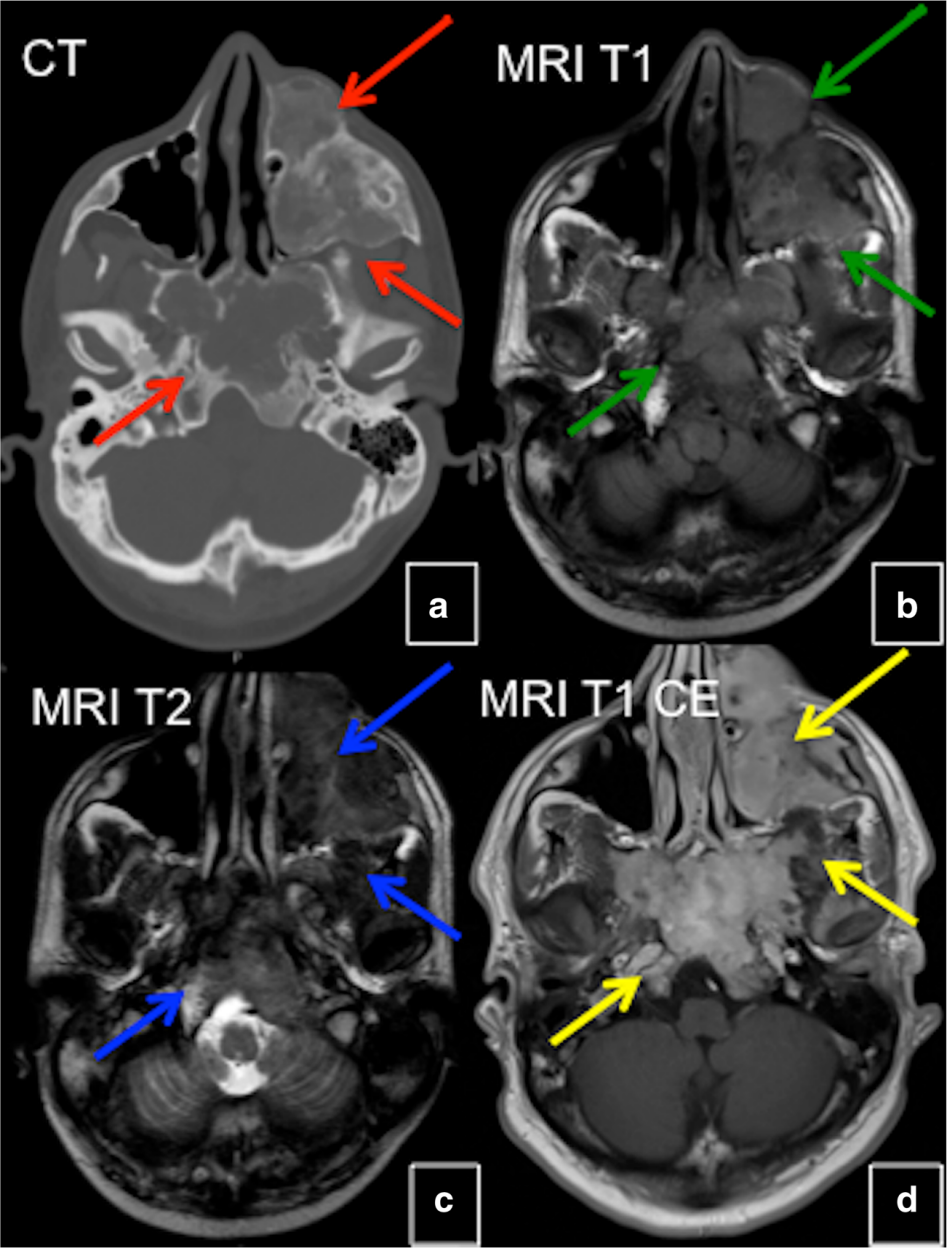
CT and MRI in craniofacial fibrous dysplasia (FD). a CT demonstrates mixed sclerotic FD lesions involving the skull base (*red arrows*). b Lesions demonstrate intermediate signal intensity on T1 weighted MRI (*green arrows*). On T2 weighted images lesions demonstrate heterogeneous hypointense/intermediate signal intensity (*blue arrows*). d FD lesions show slightly heterogeneous enhancement (*yellow arrows*)
